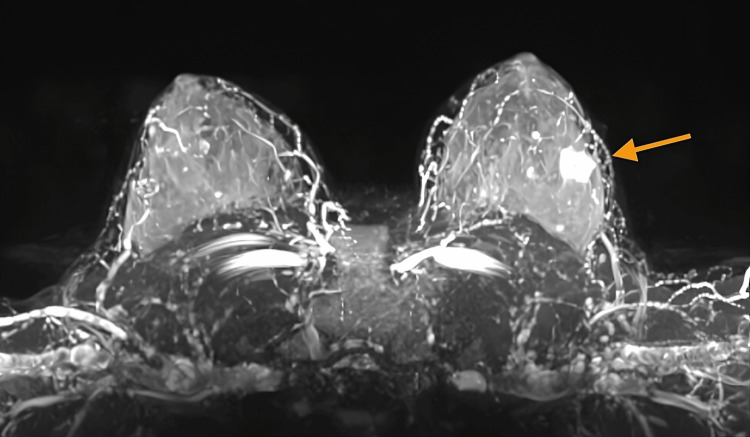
The MRI (T2WI-3D) showed a solid lesion with lobular margins located at the four o’clock position of the left breast (arrow), showing hyperintensity on T2WIs MRI: magnetic resonance imaging; T2WI: T2-weighted image; 3D: three dimensional remodeling
